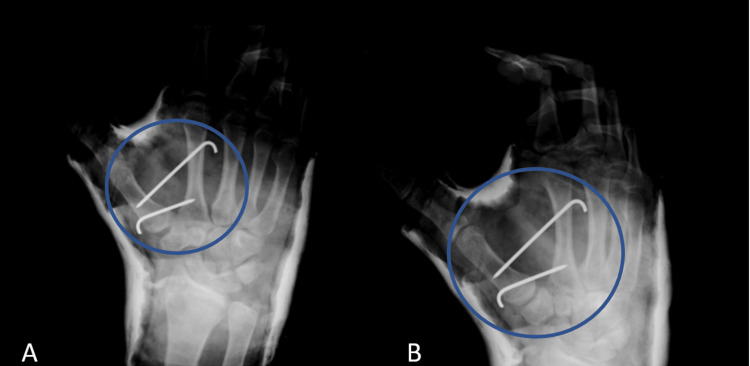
(A, B) Post operative radiographs of the hand showing a reduced trapezio-metacarpal joint that is fixed using Iselin extra-focal pinning technique.
